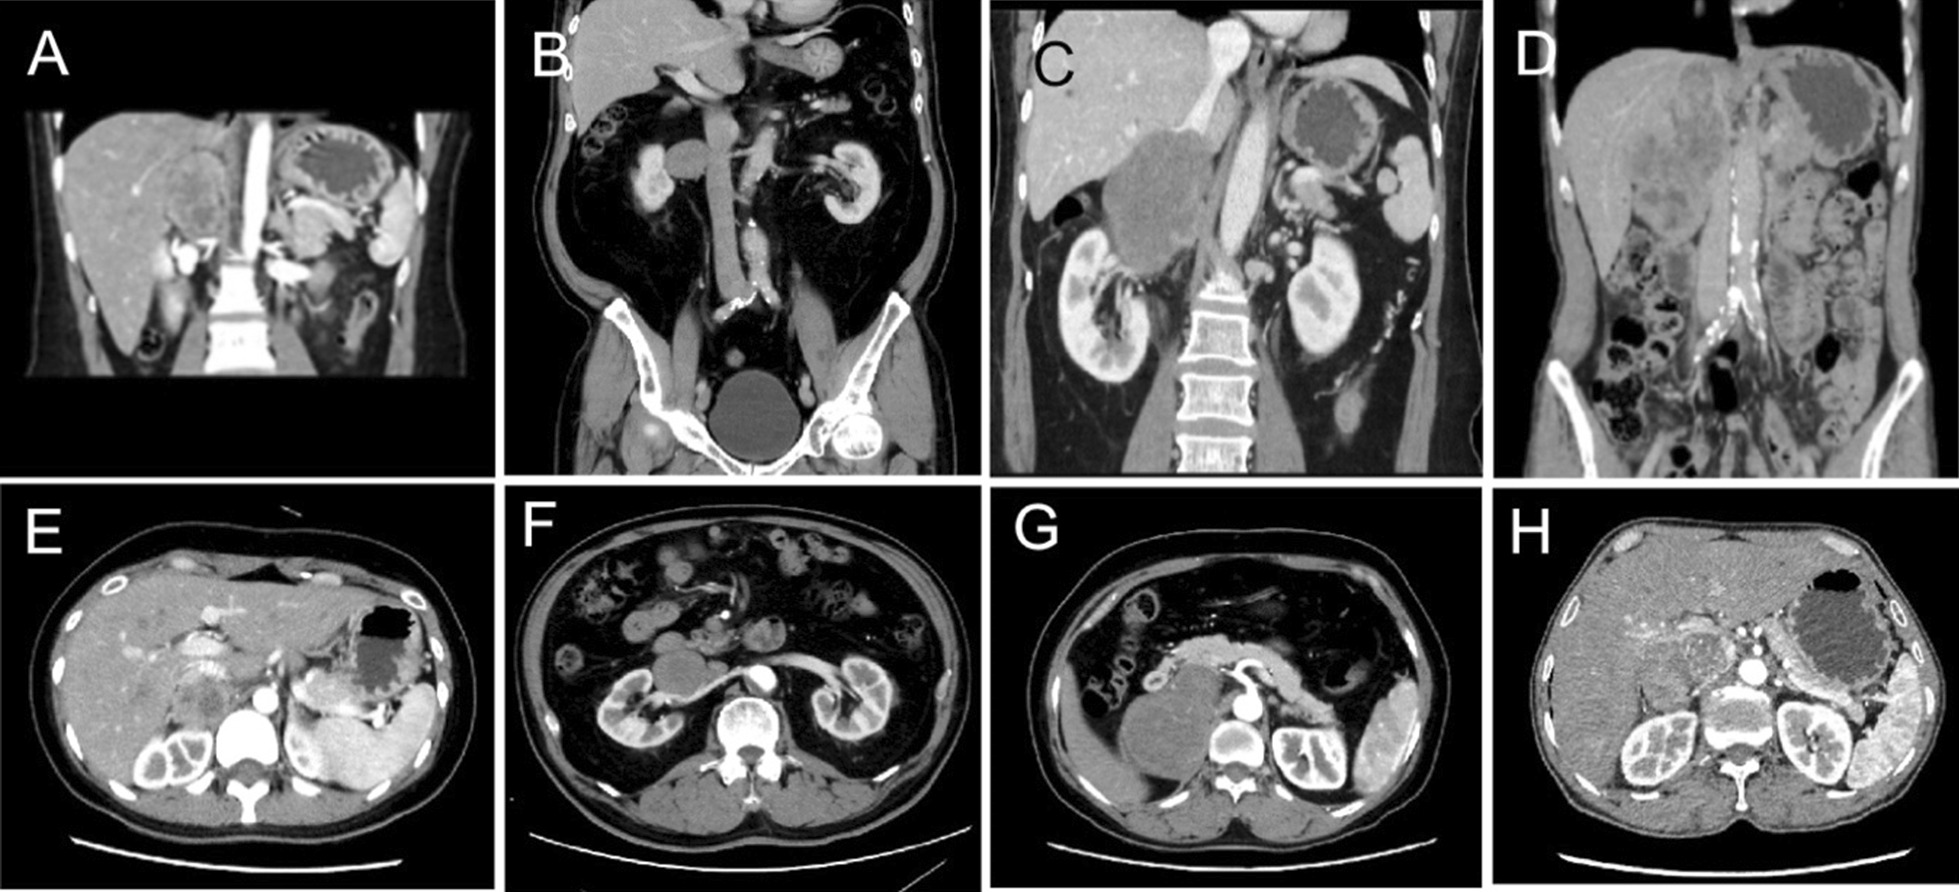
Preoperative CT images of the patients. Two patients had a mass in the right adrenal area, one patient in the right renal hilum area, and one patient in the area of the right adrenal gland anterior to the right kidney. A/E case 1, B/F case 2, C/G case 3; D/H case 4
